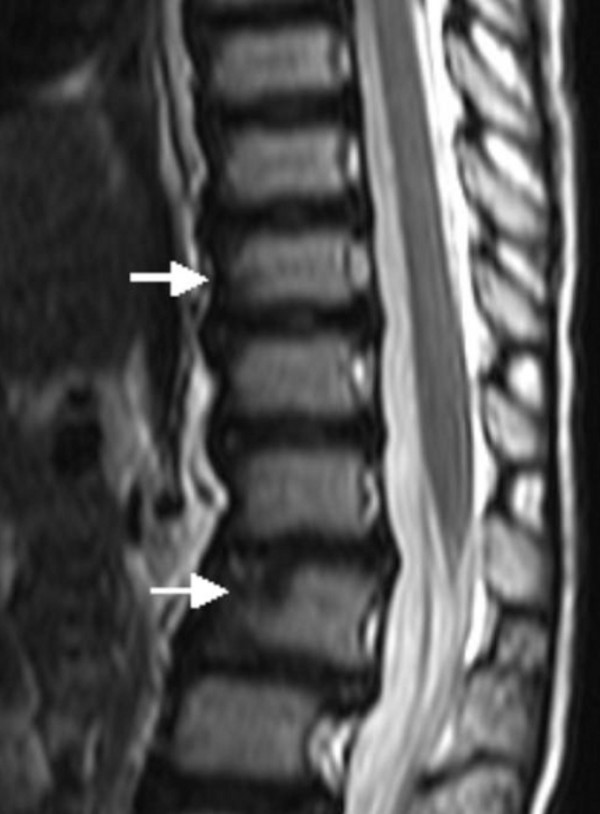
Sagittal MRI imaging of the spine showed platyspondyly associated with anterior clefting of the 12 Th thoracic vertebra (arrow) and hook-like deformity of the 5 Th lumbar vertebra (fig 4).
